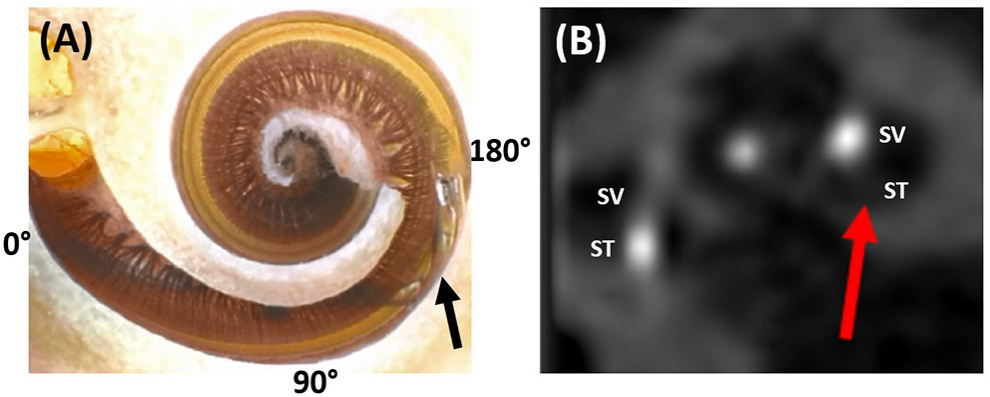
Dissected cochlear sample showing the electrode tip of a pre-shaped electrode penetrating the spiral ligament from the scala tympani (ST) and translocating to scala vestibuli (SV) (A). Image courtesy of Prof. Peter Roland from Southwestern University, TX, USA. Post-operative CT image slice showing part of the electrode in the SV as pointed by the red arrow (B). Reproduced by permission of Elsevier B.V (Appendix 2—reference 2).
